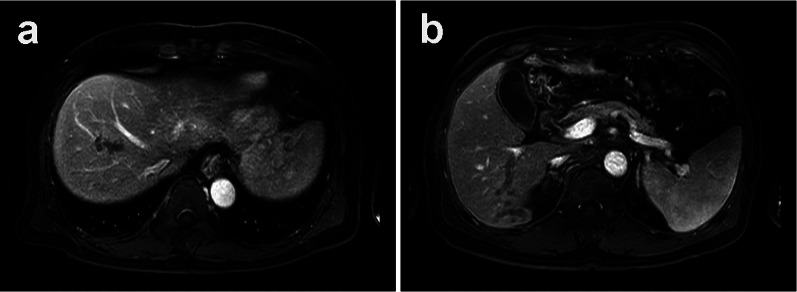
Ethoxybenzyl diethylenetriamine pentaacetic acid-enhanced magnetic resonance imaging (EOB-MRI). At the portal venous phase, the portal vein was cut off in the middle. Dilated vessels are observed from the obstruction to peripheral sites, indicating PVTT. a S8, b S6/7
